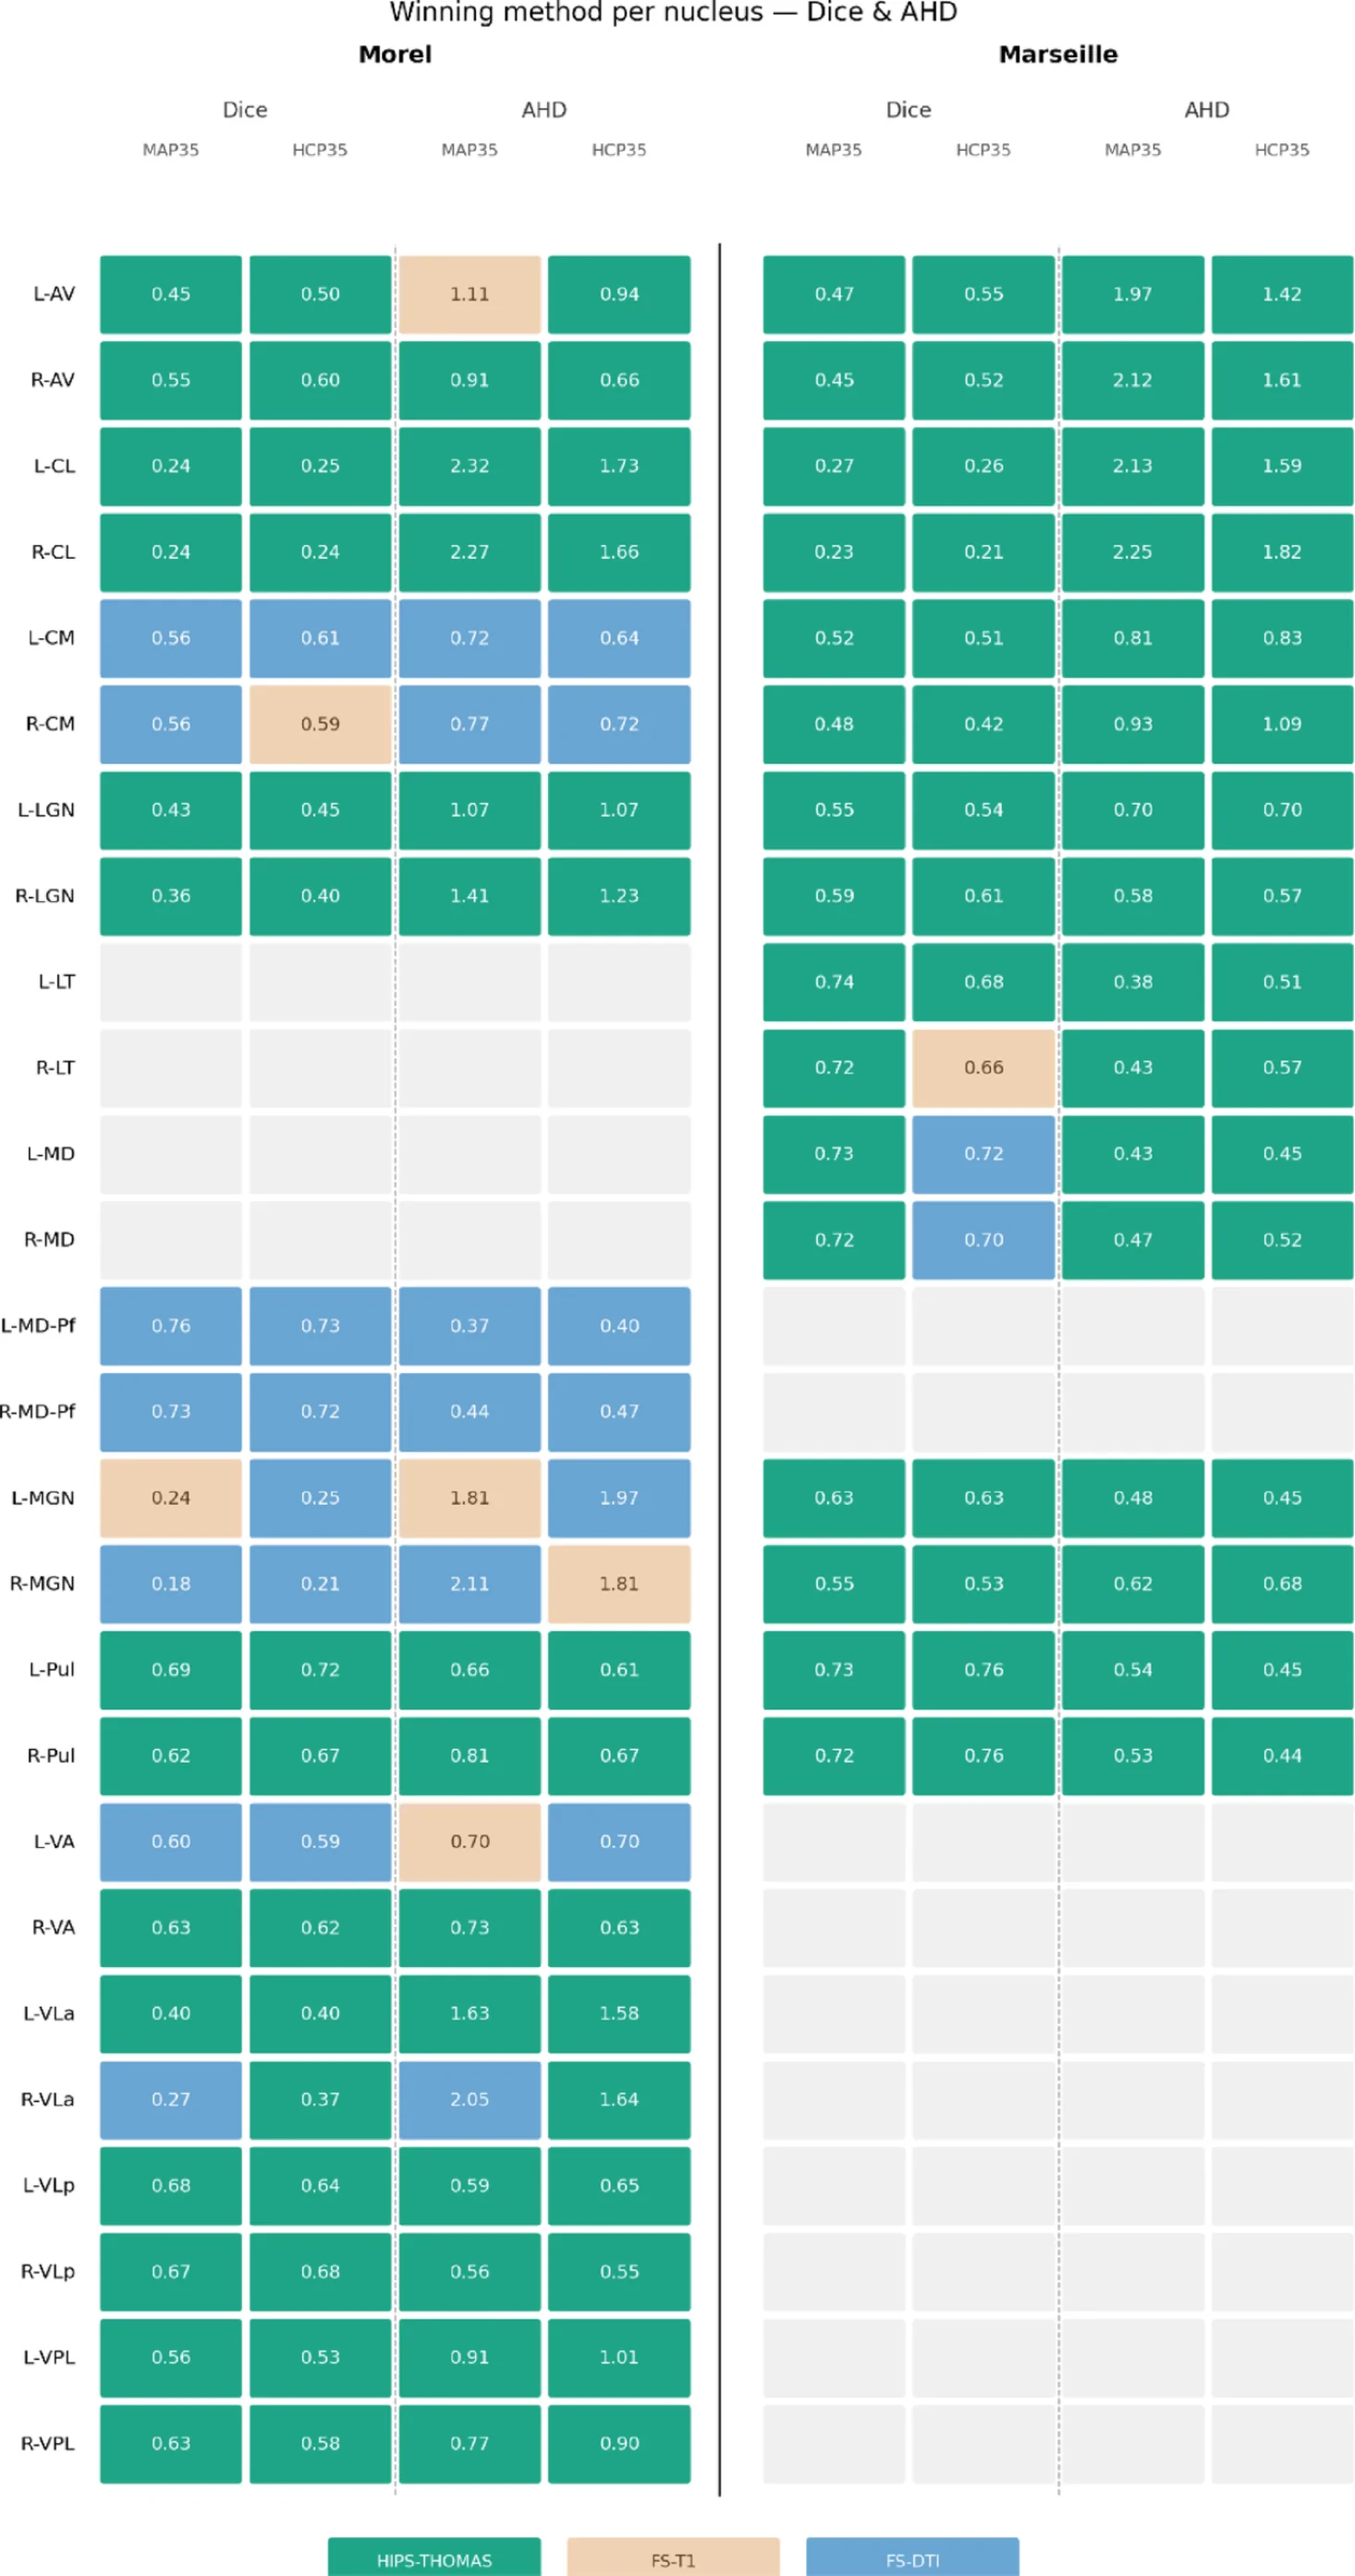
“Winner Map” of Dice coefficients and AHD for nuclei segmented with FS-T1, FS-DTI, and HIPS-THOMAS relative to Morel and Marseille reference segmentations (native space; see also Supplementary Figs. 1–4); AV: anteroventral nucleus; LT: lateral nuclei (Marseille); VA: ventral anterior nucleus; VLa: Ventrolateral anterior nucleus; VLp: Ventrolateral posterior nucleus; MD-Pf: mediodorsal-parafascicular nuclei; Pul: pulvinar nucleus; VPL: Ventral Posterolateral nucleus; CL: Centrolateral nucleus; CM: Centromedian nucleus; LGN: Lateral Geniculate Nucleus; MGN: Medial Geniculate Nucleus; L/R: Left, Right hemisphere; FS-T1: T_1_-based FreeSurfer segmentation (Iglesias et al. 2018); FS-DTI: FreeSurfer’s joint segmentation of thalamic nuclei from T1 scan and DTI (Tregidgo et al. 2023); (Vidal et al. 2024); AHD: Average Hausdorff Distance; HIPS-THOMAS: Thalamus Optimized Multi-atlas Segmentation using Histogram-based Polynomial Synthesis (Vidal et al. 2024); MAP35, HCP35: datasets with T1w-MRIs and diffusion MRI available; black diamond: mean; black horizontal line: median; Morel: Krauth-Morel atlas (Krauth et al. 2010); Marseille: the custom-made MNI version of the atlas of deep grey matter nuclei, part of the 7TAMIbrain dataset (Brun et al. 2022)
